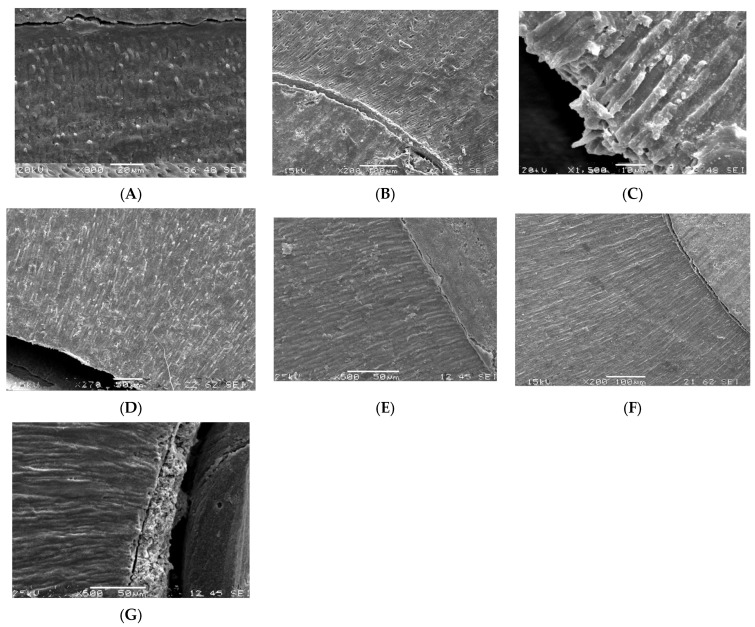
(A). Group 1: short resin tags (G2 Bond) are visible and adhesive failure type (SEM x800). (B). Group 2: no resin tags (G-Premio Bond) are visible and adhesive failure type (SEM x200). (C). Group 3: Resin tags (G2 Bond) formation after ultrasonic activation (SEM x1200). (D). Group 4: Resin tags (G-Premio Bond) after ultrasonic activation and adhesive failure (SEM x270). (E). Group 5: Mixed failure (SEM x500). (F). Group 6: Resin tags formation (G-Premio Bond) after ultrasonic activation and adhesive failure (SEM x300). (G). Group 7: Mixed failure sample; the SEM picture shows the debonding at cement and post interface (SEM x500).
